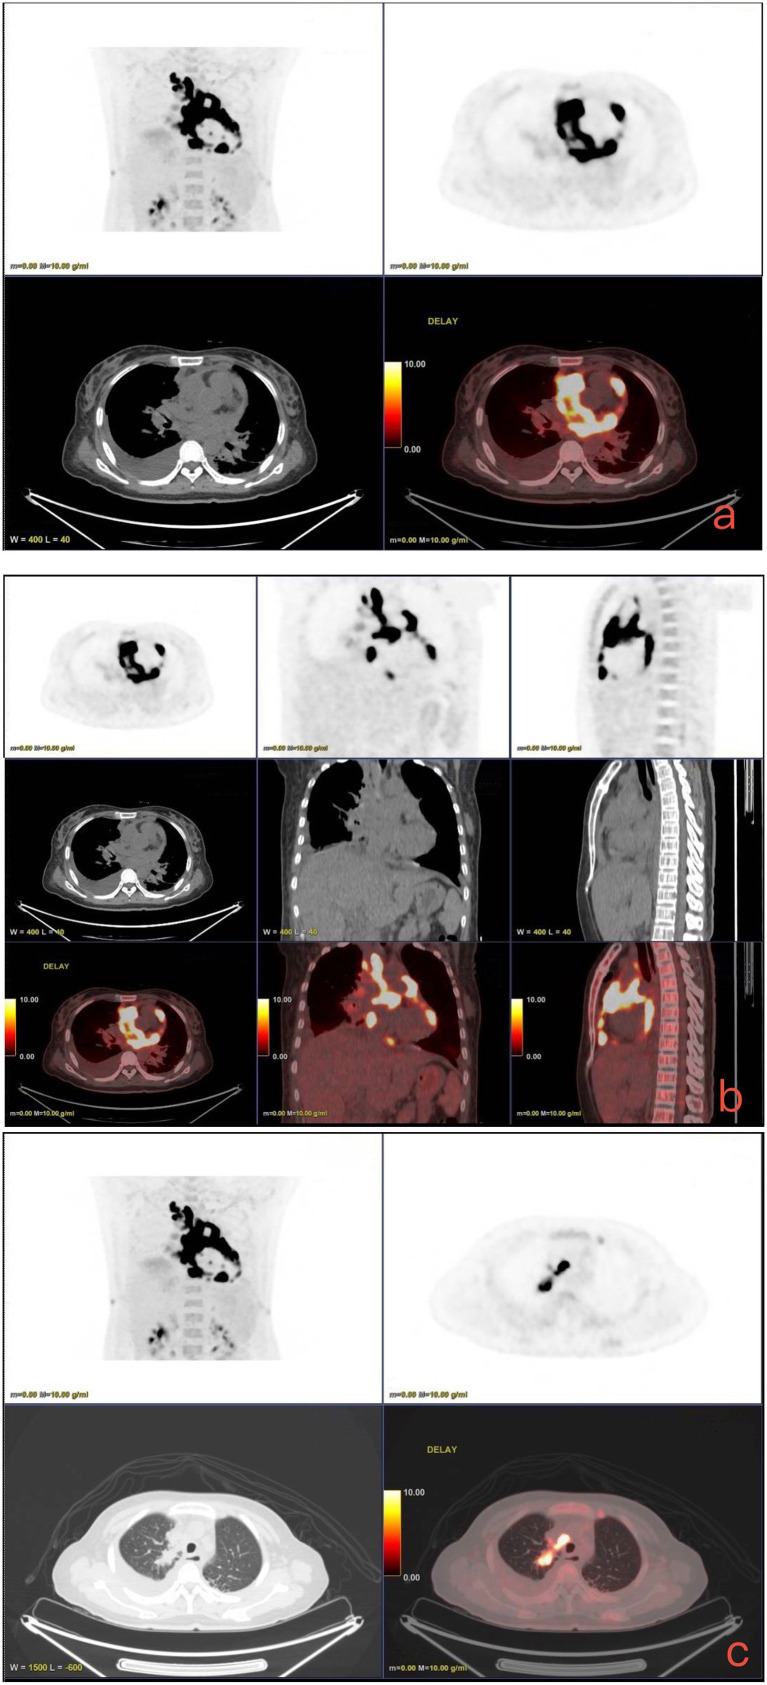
PET/CT (November 18, 2024). (a–c) Show uneven enhancement of the pericardium and mediastinal soft tissues with increased glucose metabolism, highly suggestive of a specific infectious lesion.
